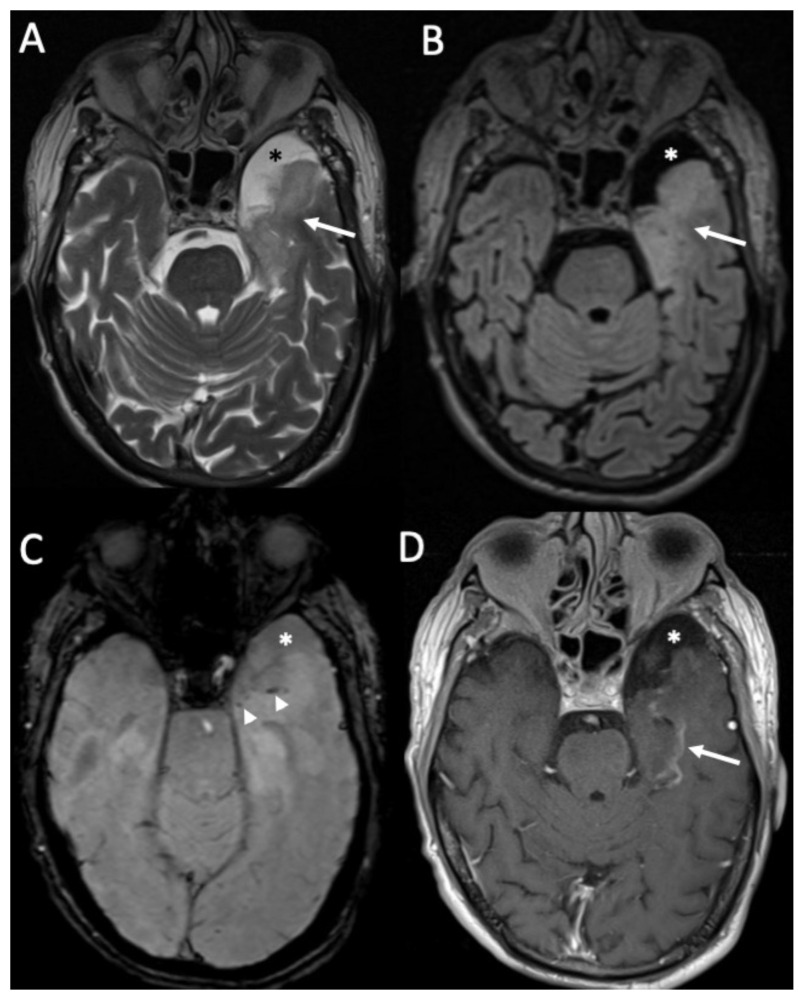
Axial T2 (A), FLAIR (B), susceptibility-weighted imaging (C), and enhanced T1 (D) show hyperintensity of the temporal lobe on T2 and FLAIR (arrows in A,B) in a patient with Herpes virus simplex type 1 encephalitis in the subacute phase. There are also some hemorrhagic foci (arrowheads in C) and contrast enhancement (arrow D). Note also concomitant temporo-polar arachnoid cyst (asterisk in A–D).
